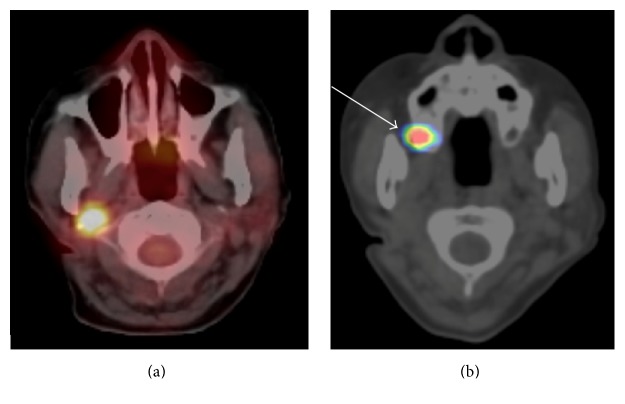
(a) ^18^F-FDG PET/CT image: remarkable abnormal FDG uptake by the lymph node metastasis in the right parapharyngeal space. (b) ^131^I SPECT/CT image obtained 7 days after a ^131^I 3.7 GBq dose of RIT: ^131^I uptake by the lymph node metastasis is not evident. The abnormal distribution in the right maxillary sinus (white arrow) represents ^131^I secretion into the nasal fluid.
